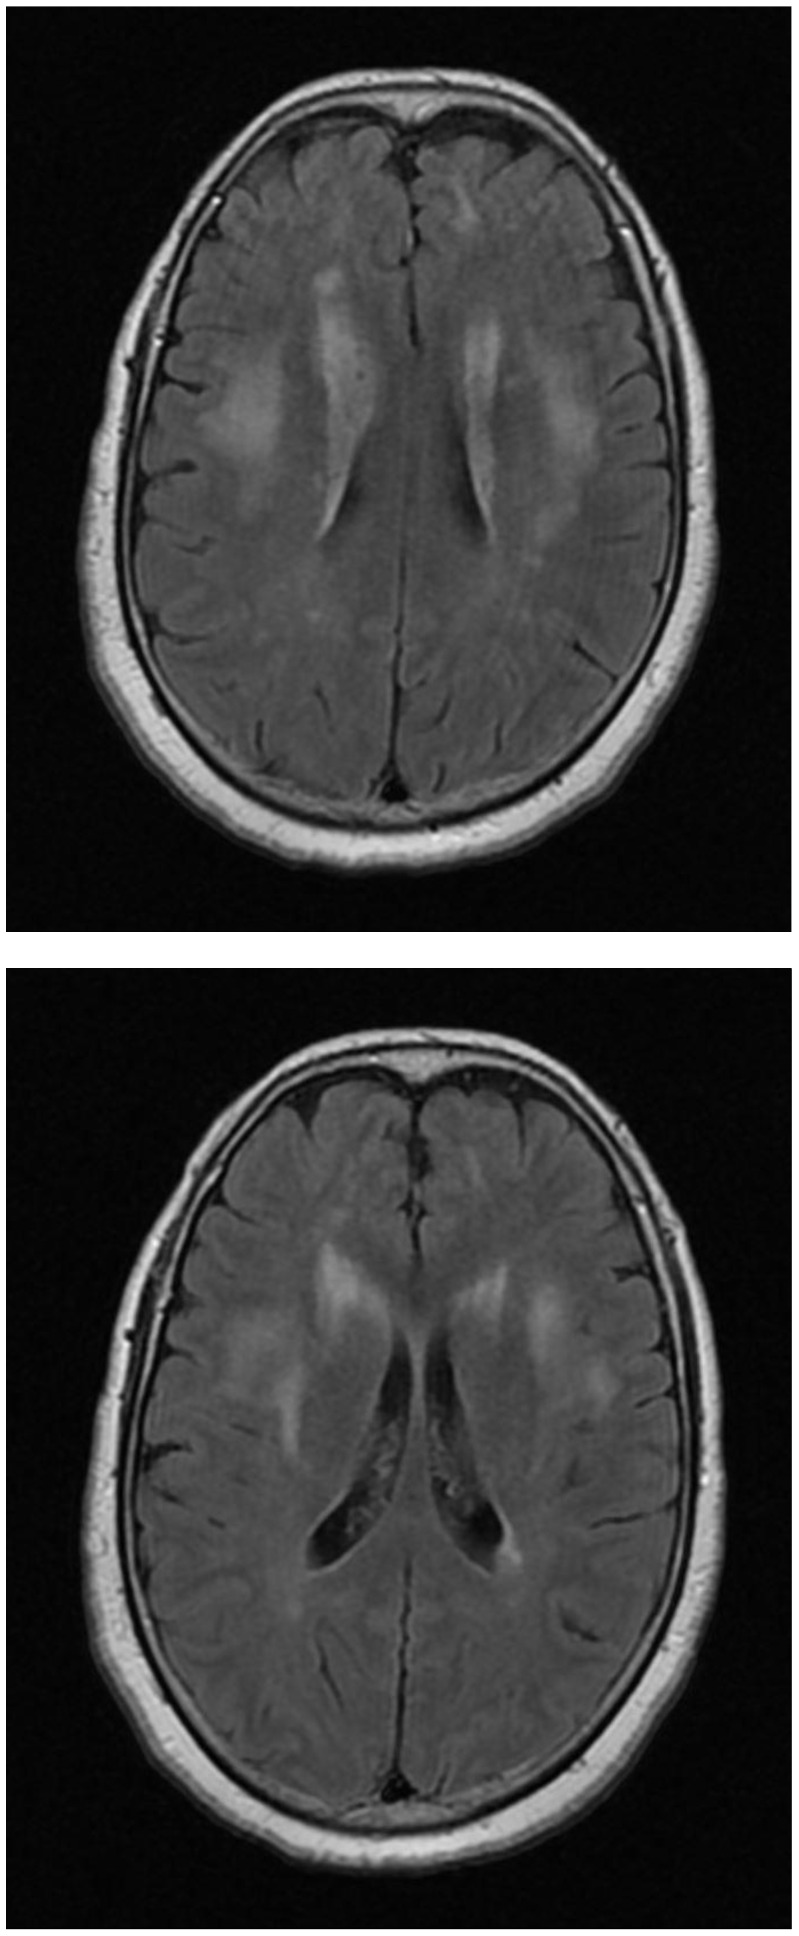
MRI of brain: fluid-attenuated inversion recovery (FLAIR) sequences, showing multifocal, bilateral, asymmetric, multiple hyperintense lesions in the deep and subcortical white matter. The thalami and basal ganglia.
